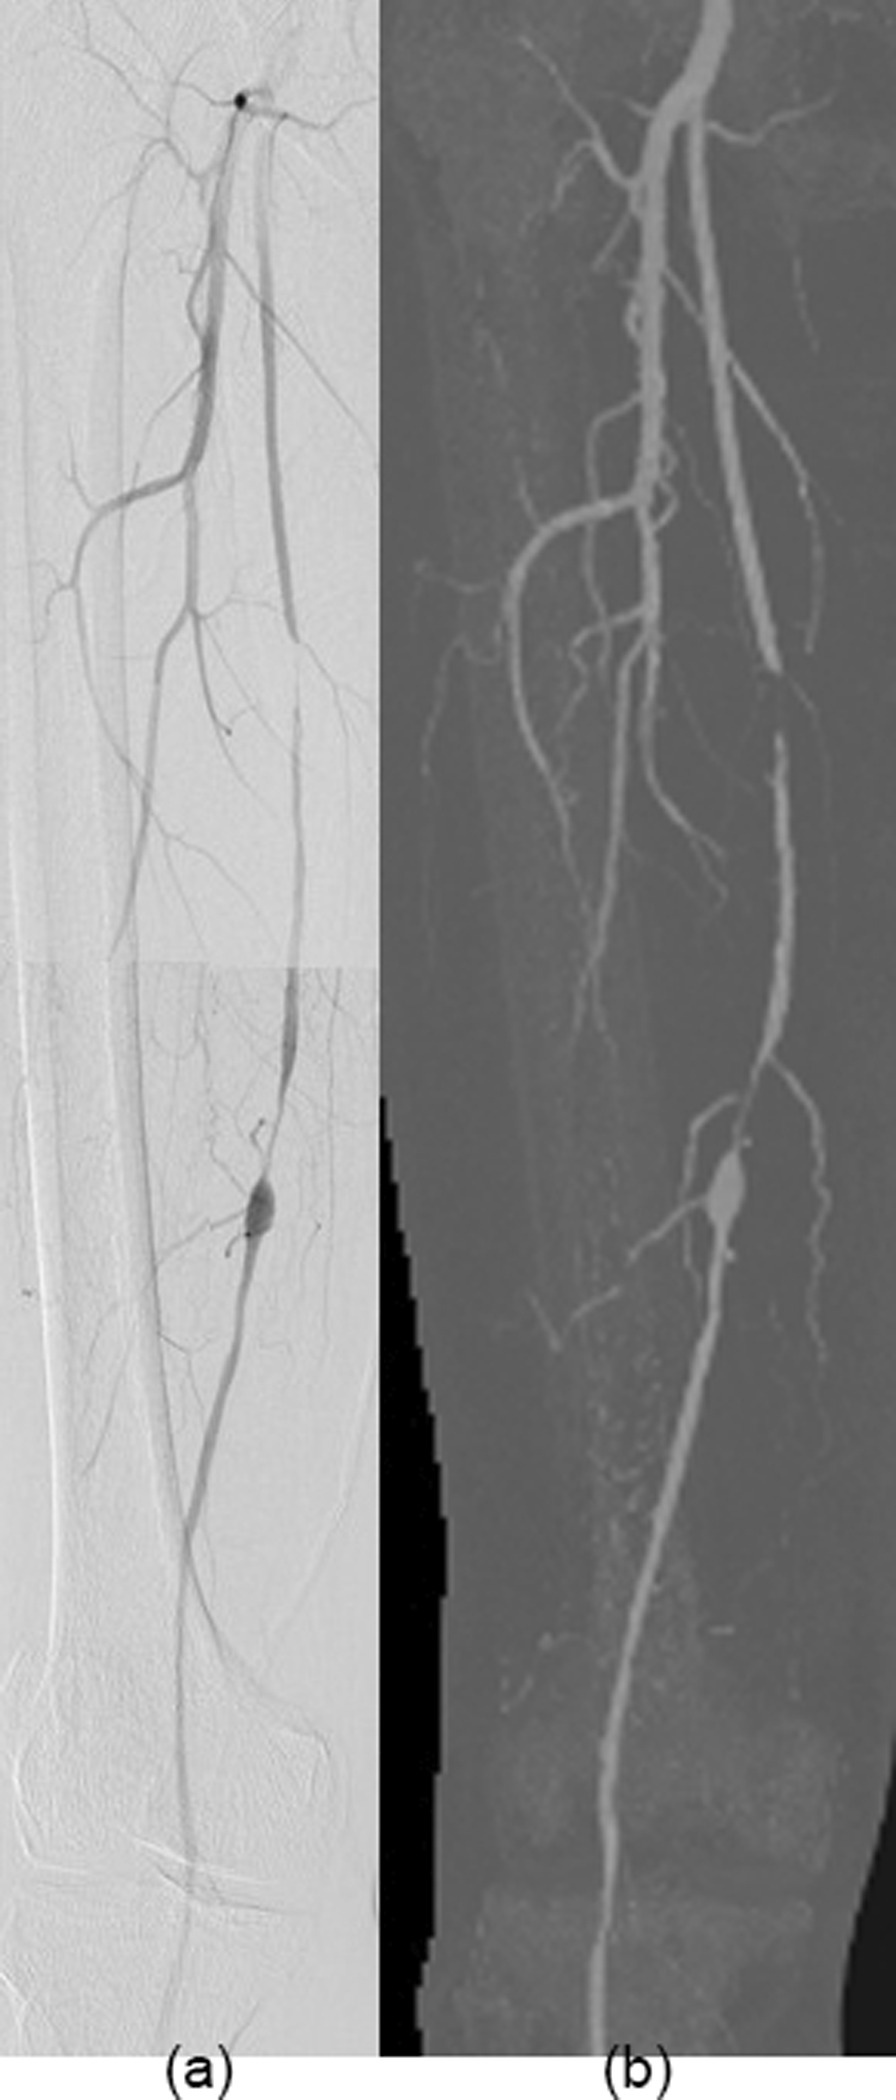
A 67-year-old male with intermittent claudication of the right lower limb for 1 month. There was occlusion and significant stenosis in the proximal and middle superficial femoral arteries, respectively. An aneurysmal dilatation was found immediately after significant stenosis. DSA was performed within twenty-nine days. Panel a shows the DSA image and Panel b shows the maximum intensity projection CTA image
